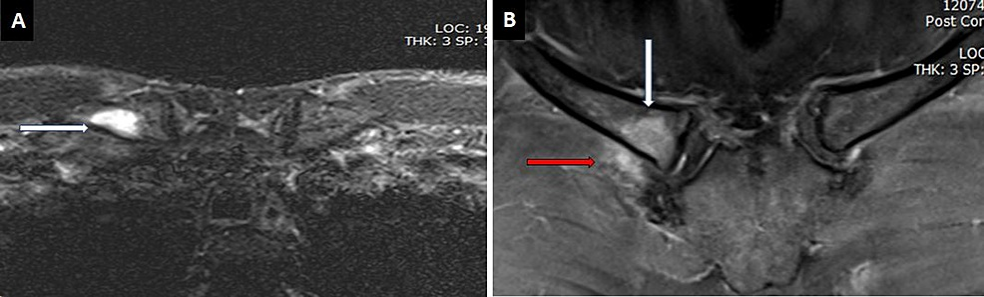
MRI of the sternoclavicular joint A. Selected axial T2-weighted image showing subchondral bone marrow edema (white arrow). B. T1-weighted gadolinium-enhanced image demonstrating enhancement in the medial end of the right clavicle (white arrow) with mild effusion of the right sternoclavicular joint, and surrounding soft tissue edema and enhancement (red arrow) MRI: magnetic resonance imaging
